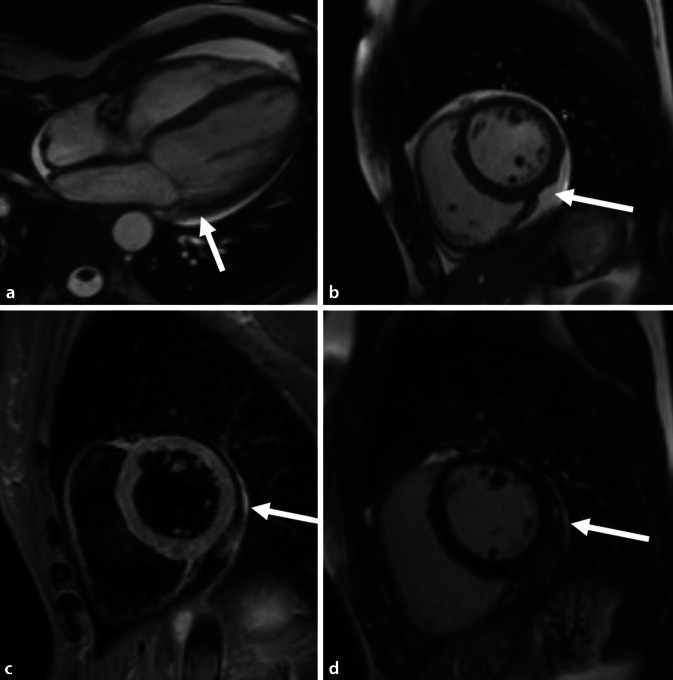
Die Pathophysiologie der Perikarditis wird in erster Linie als Beteiligung im Rahmen einer Myokarditis gesehen. Bildgebend wird die Perikarditis anhand einer Perikardverdickung zusammen mit einer perikardialen Kontrastmittelaufnahme in der MRT nachgewiesen. Daneben kann auch die Durchführung einer Positronenemissionstomographie (PET) oder eine CT des Herzens hilfreich sein.
